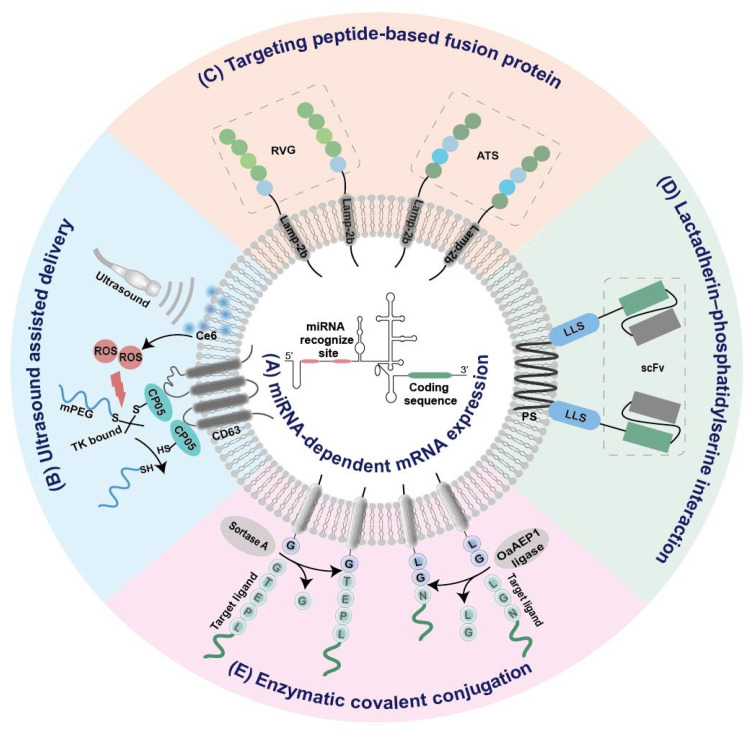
Summary of EV-based platforms for tissue-specific mRNA expression. (A) miRNA-dependent mRNA expression. (B) Ultrasound assisted delivery. (C) Targeting peptide-based fusion protein. (D) Lactadherin–phosphatidylserine interaction. (E) Enzymatic covalent conjugation. IRES, internal ribosome entry site; RVG, rabies viral glycoprotein; ATS, adipocyte-targeting sequence; scFv, single chain variable fragment; LLS, lactadherin leader sequence; Ce6, sonosensitizer chlorin e6; ROS, reactive oxygen species; PS, phosphatidylserine; TK, thioketal.
